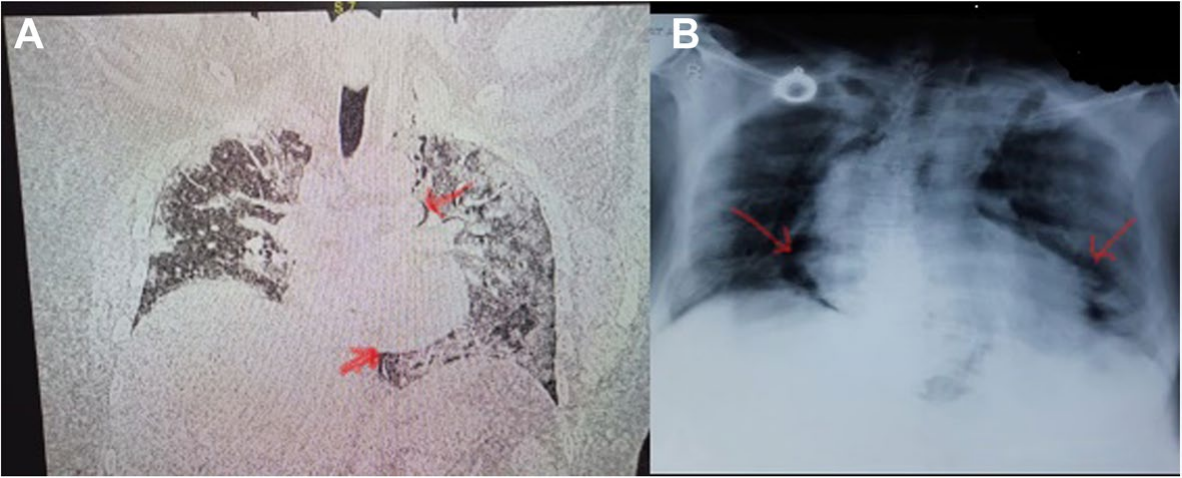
A HRCT on admission. B CXR showing pneumomediastinum (red arrows)
